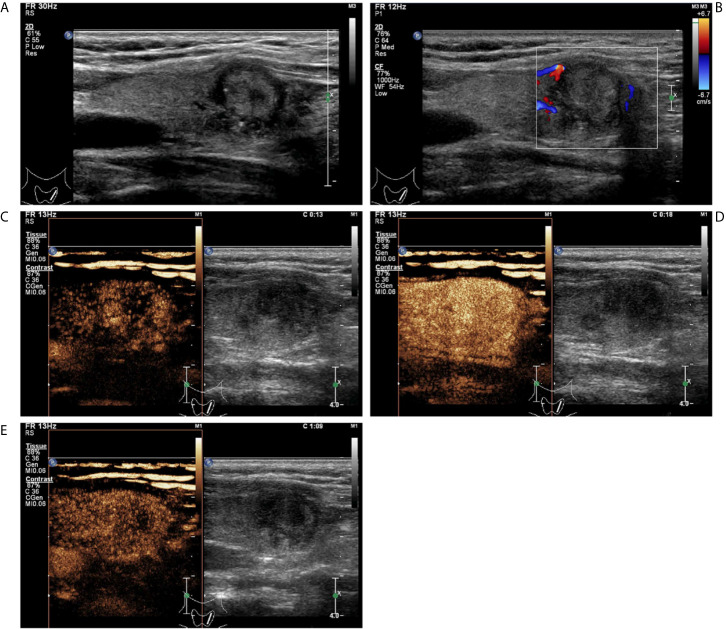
A follicular variant of papillary thyroid carcinomas in a 53-year-old woman. (A) Greyscale ultrasound showed that there was a solid isoechoic nodule in the left lobe of the thyroid, with irregular hypoechoic halo, irregular margin, wider-than-tall shape, and punctate echogenic foci. The nodule was ACR TI-RADS category 5. (B) Color Doppler showed peripheral vascularity. (C) Contrast-enhanced ultrasound revealed the trend that contrast agent enters the nodule from the periphery to the center (centripetal enhancement) at the time of the 13th second after the injection of contrast agent. (D) Contrast-enhanced ultrasound revealed heterogenous hyper-enhancement at peak (the 18th second after the injection of contrast agent), without a regular enhanced ring. (E) Contrast-enhanced ultrasound revealed heterogenous hypo-enhancement within the nodule at the 69th second after the injection of contrast agent, which indicates earlier wash-out than the surrounding thyroid parenchyma.
